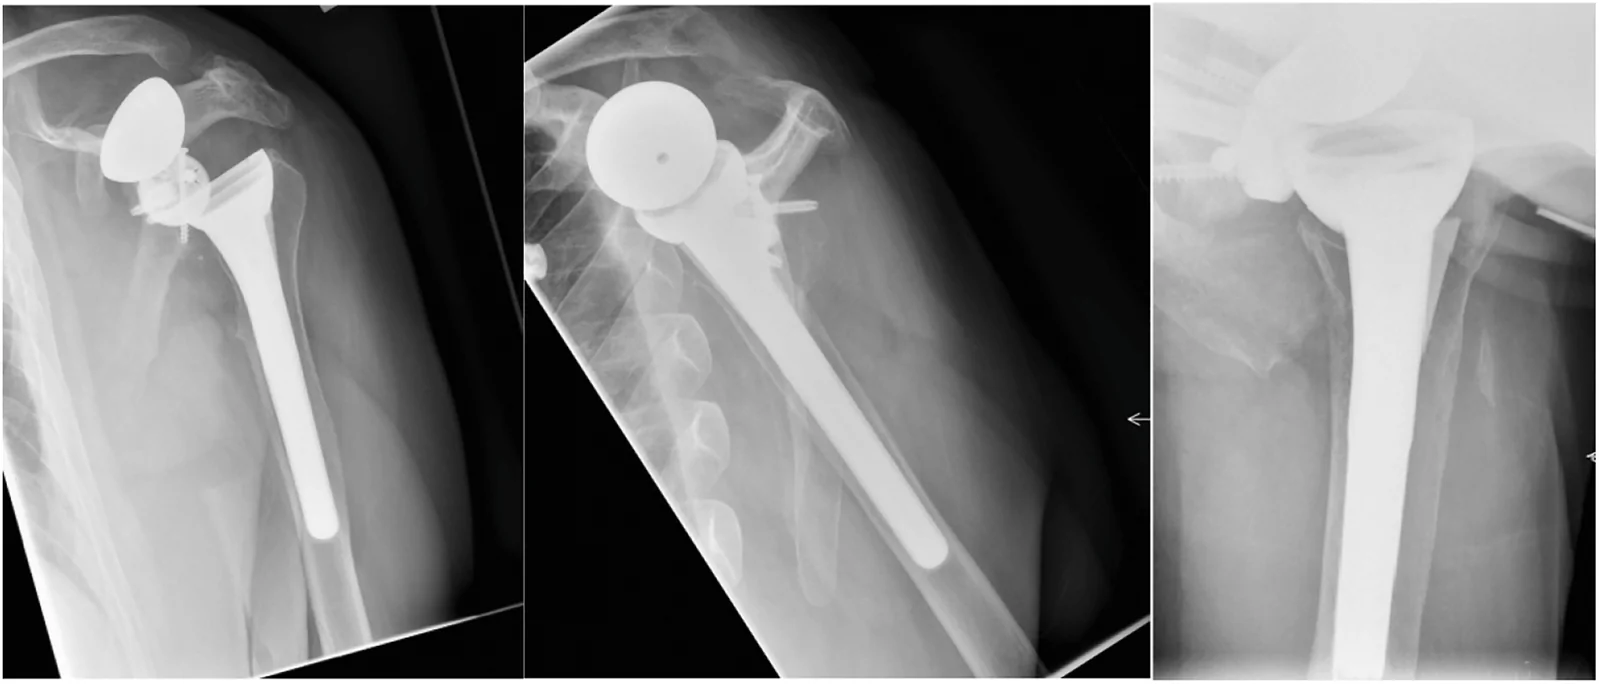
Case 2 x-rays showing complete glenosphere disengagement.
